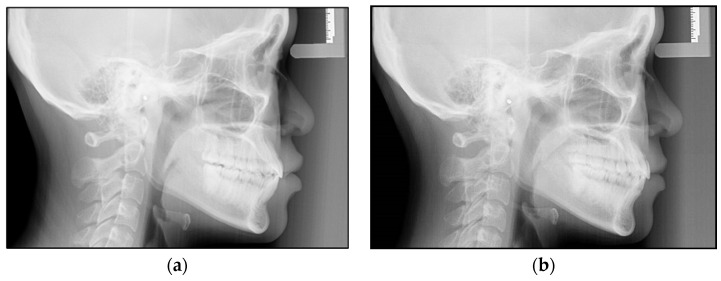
(a,b) Lateral cephalometric radiographs of a patient at the beginning of the treatment (a) and after treatment with the first series of Invisalign (b).
